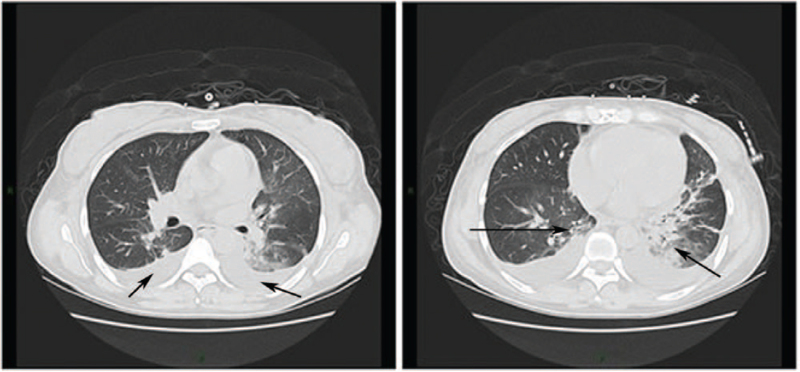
Chest CT on Day 12. Bilateral ground-glass opacity, atelectasis in the lower lobes of both lungs, especially in the left lung. Bilateral pleural effusion and a small amount of interlobar effusion on the left side.
